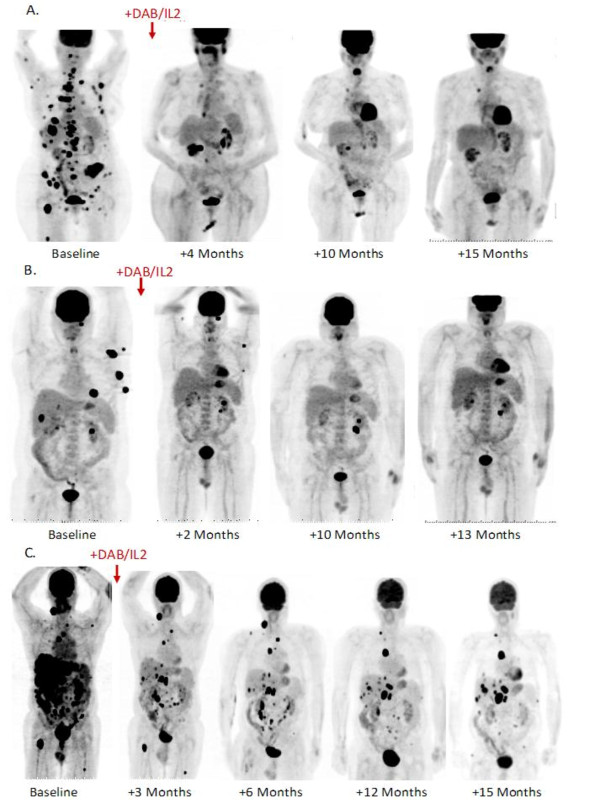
Durable responses after DAB/IL2 administration. On the left, baseline anterior/posterior views of FDG-PET imaging reveal multiple FDG-avid melanoma metastases in three clinical examples: A, 78 year-old female; B, 47 year-old male; and C, 62 year-old male. After DAB/IL2 administration, we observed a clinically significant and durable reduction in tumor burden, including hepatic metastases, pulmonary nodules, metatastic lymph nodes and subcutaneous nodules in all three patient examples.
